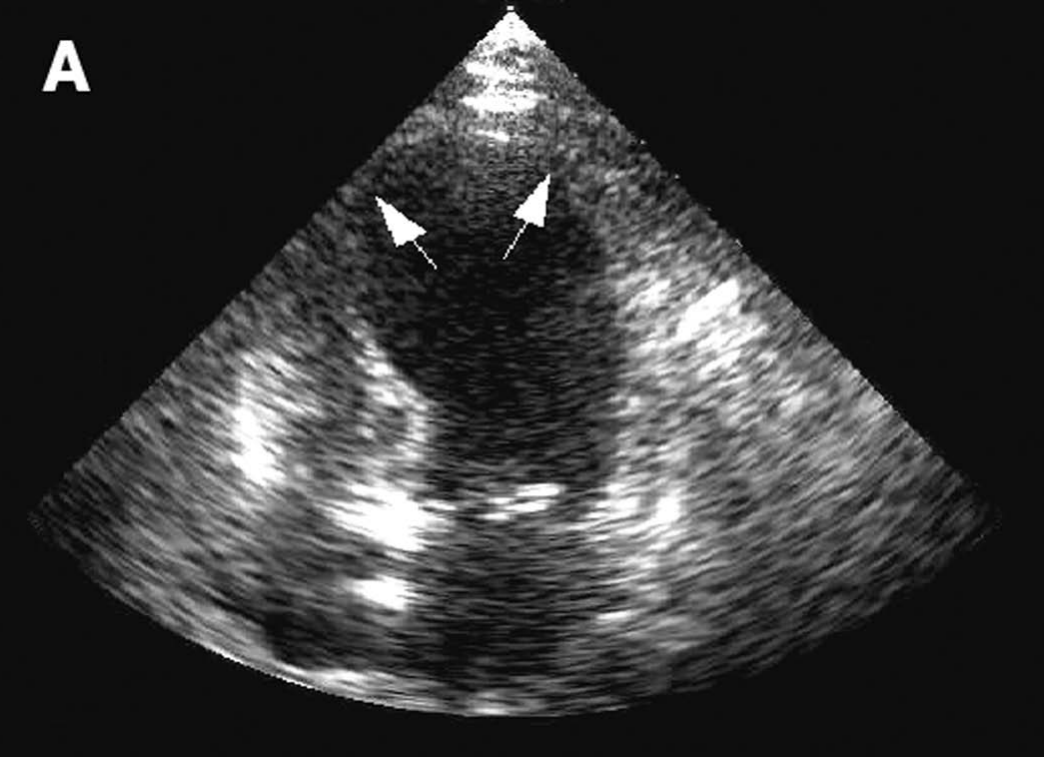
Echocardiograph showing dilatation of the left ventricle in the acute phase.
